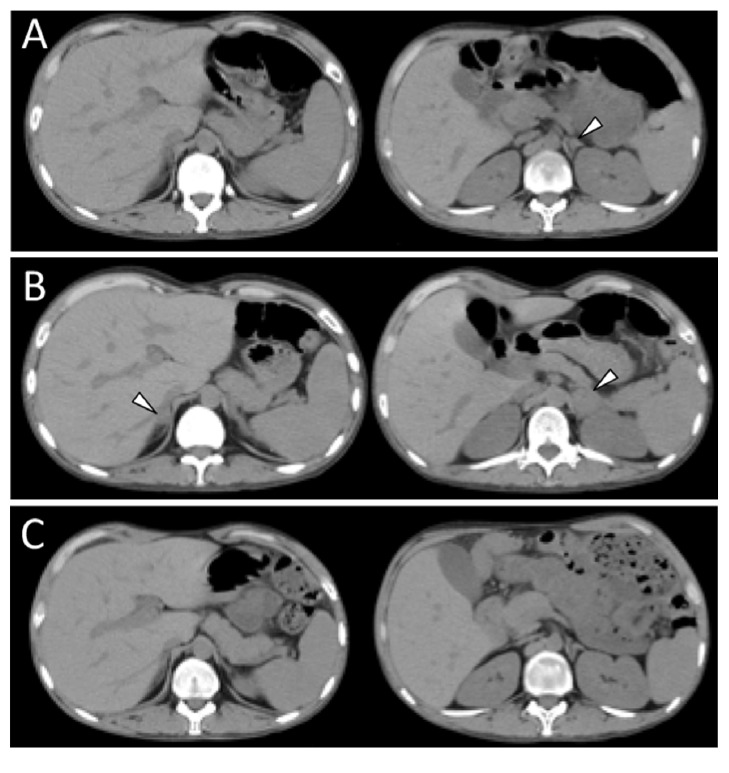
Computed tomography showing the time-course changes in the enlarged adrenal glands. Computed tomography showing gradual exacerbation of the bilaterally enlarged adrenal glands (arrowheads) evaluated at 5 months (A) and day 0 (B) before treatment and improvement at 6 months (C) after initiation of treatment.
